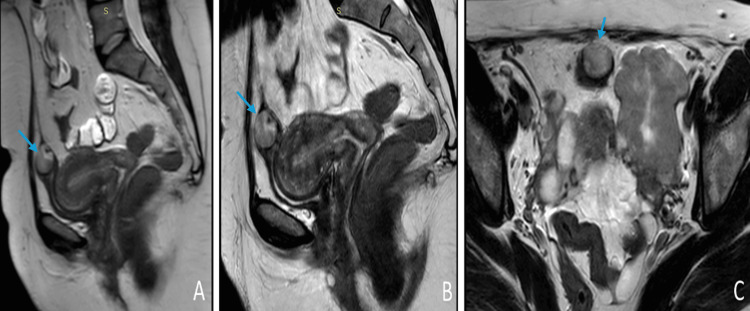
MRI of the pelvis. A, B, and C denote the presence of a cystic mass (urachal cyst) in the anterior abdominal wall (as indicated by arrows).
